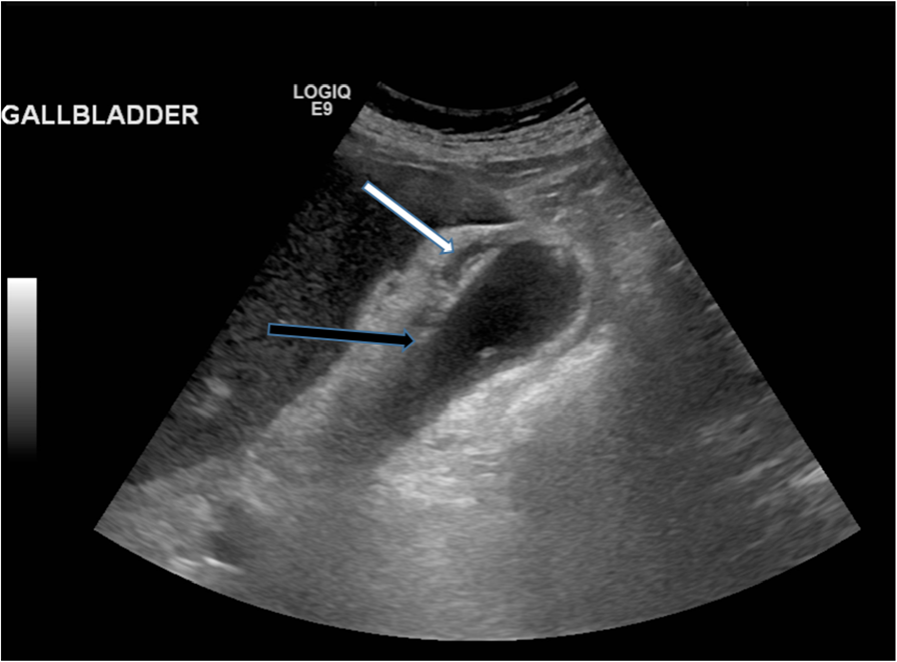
Sagittal ultrasound image of the gallbladder which contains hyperechoic gallstones. There is gallbladder wall thickening (black arrow) and pericholecystic fluid (white arrow) consistent with acute calculous cholecystitis
